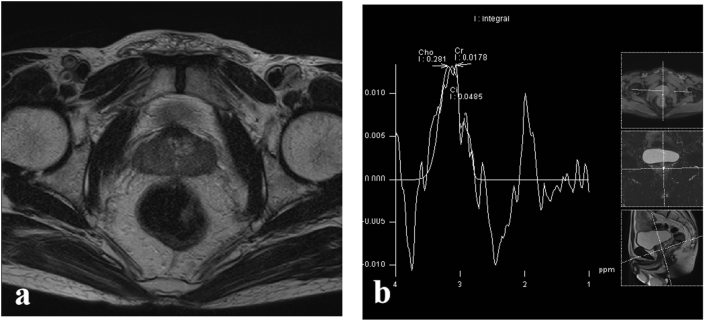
(a) T2-weighted image showing peripheral gland was of low-signal intensity. (b) MRS showing an elevated Cho peak and a reduced Ci peak in the lesion.
